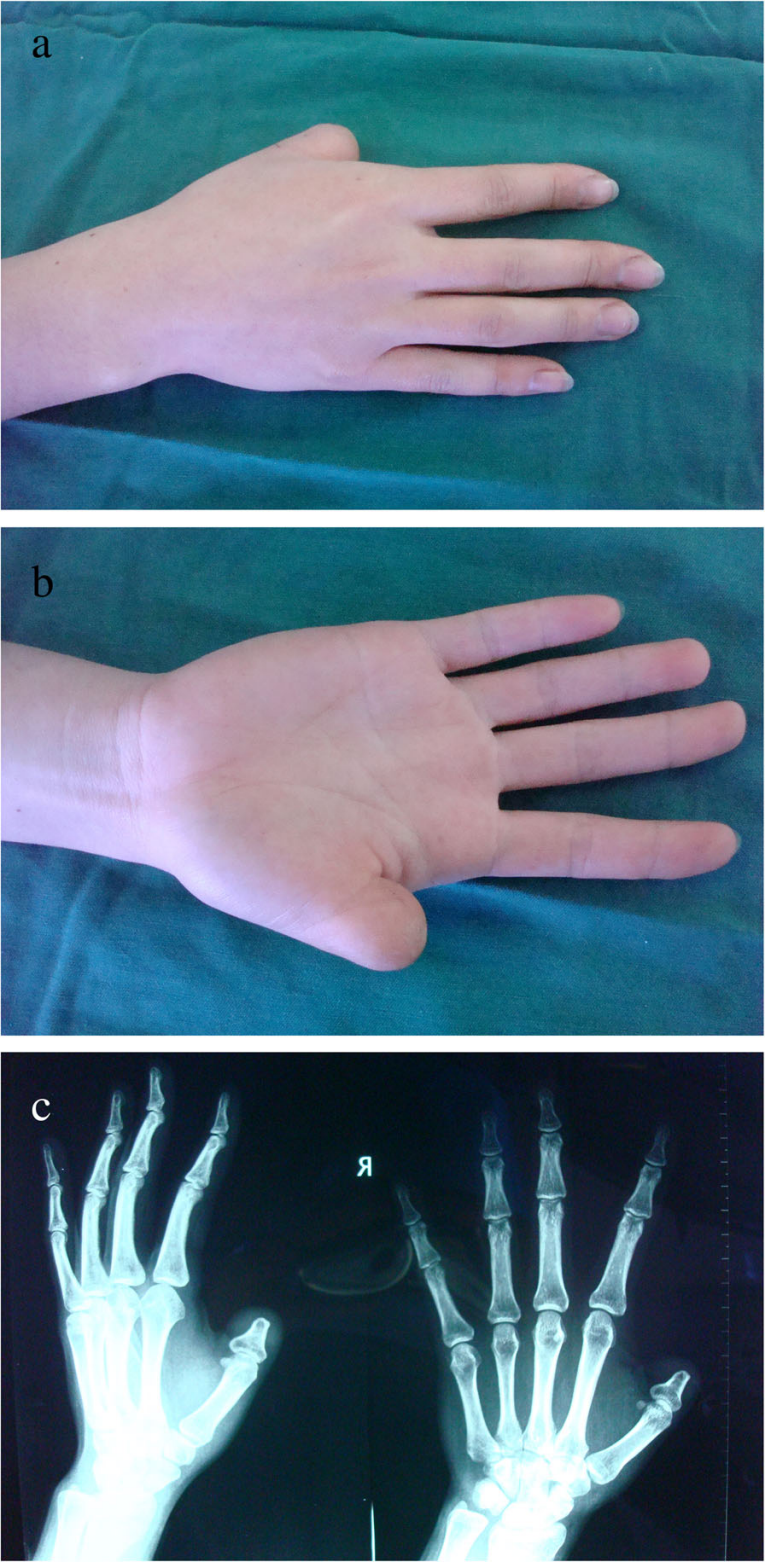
a, b Preoperative view of the right thumb defects distal to the interphalangeal (IP) joint. c Preoperative X-ray of the right hand
